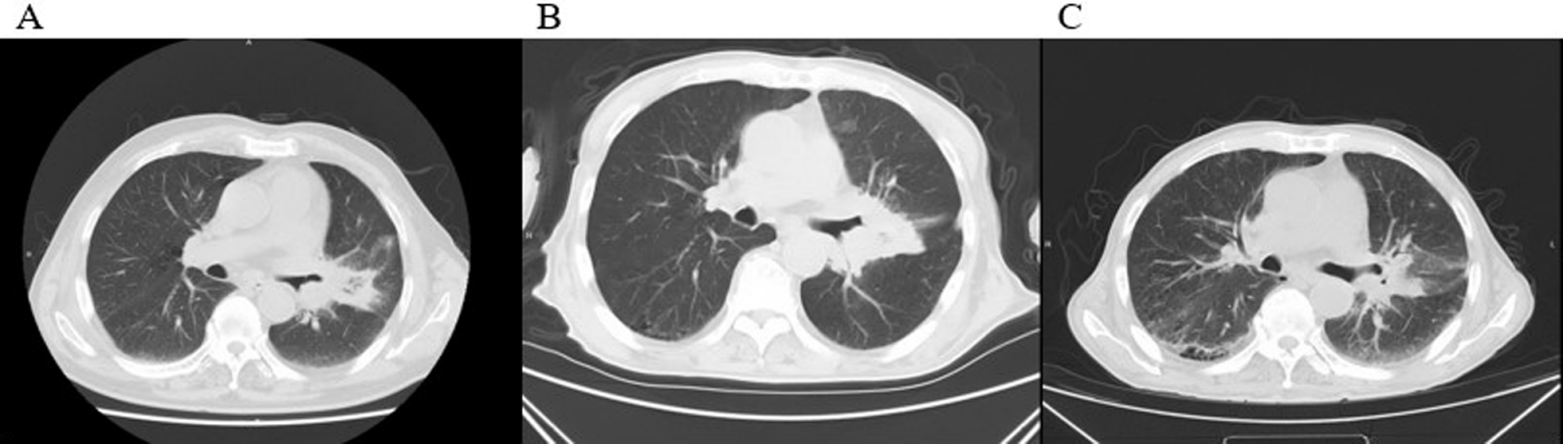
Chest CT scans (mediastinal window). A The chest CT scan showed distal obstructive inflammation, the enlargement of the left hilar and mediastinal lymph nodes when the patient was diagnosed with NSCLC. B After seven months of gefitinib treatment, the maximal tumor size in the lung lesion did not reduce. C After one month of toripalimab and bevacizumab treatment, the tumor was found to be markedly regressed
